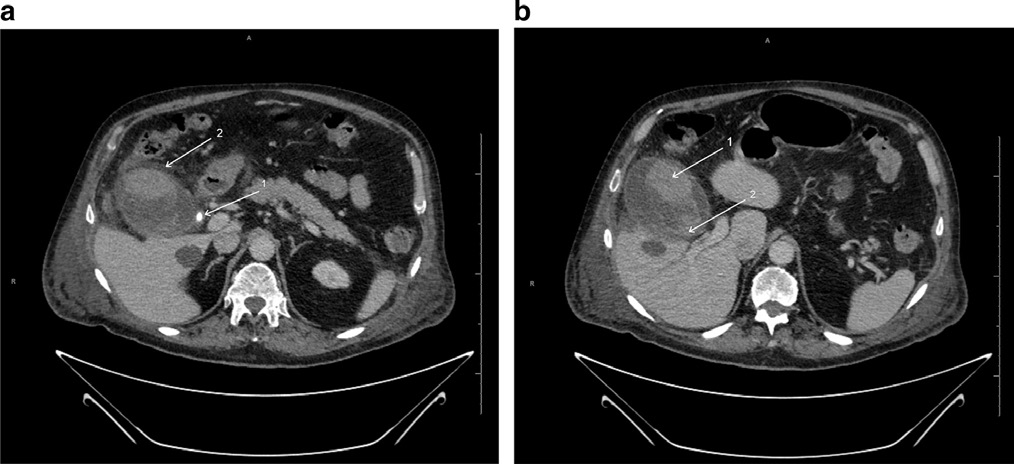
a.Contrast enhanced CT of the abdomen and pelvis. 2b. Contrast enhanced CT of the abdomen and pelvis
